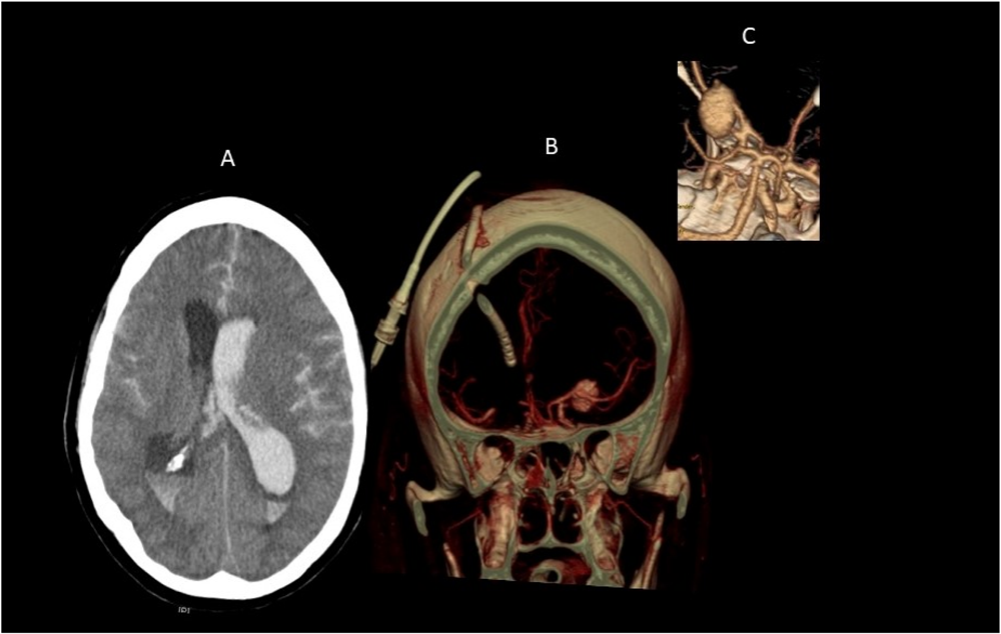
Computerised tomography (CT) of the brain and CT Angiography of subarachnoid and intraventricular haemorrhage. A Computerised tomography scan showing subarachnoid and intraventricular haemorrhage; B Culprit aneurysm shown on 3D rendering from CT angiography, treated with an external ventricular drain; C Closer view of unruptured aneurysm.
